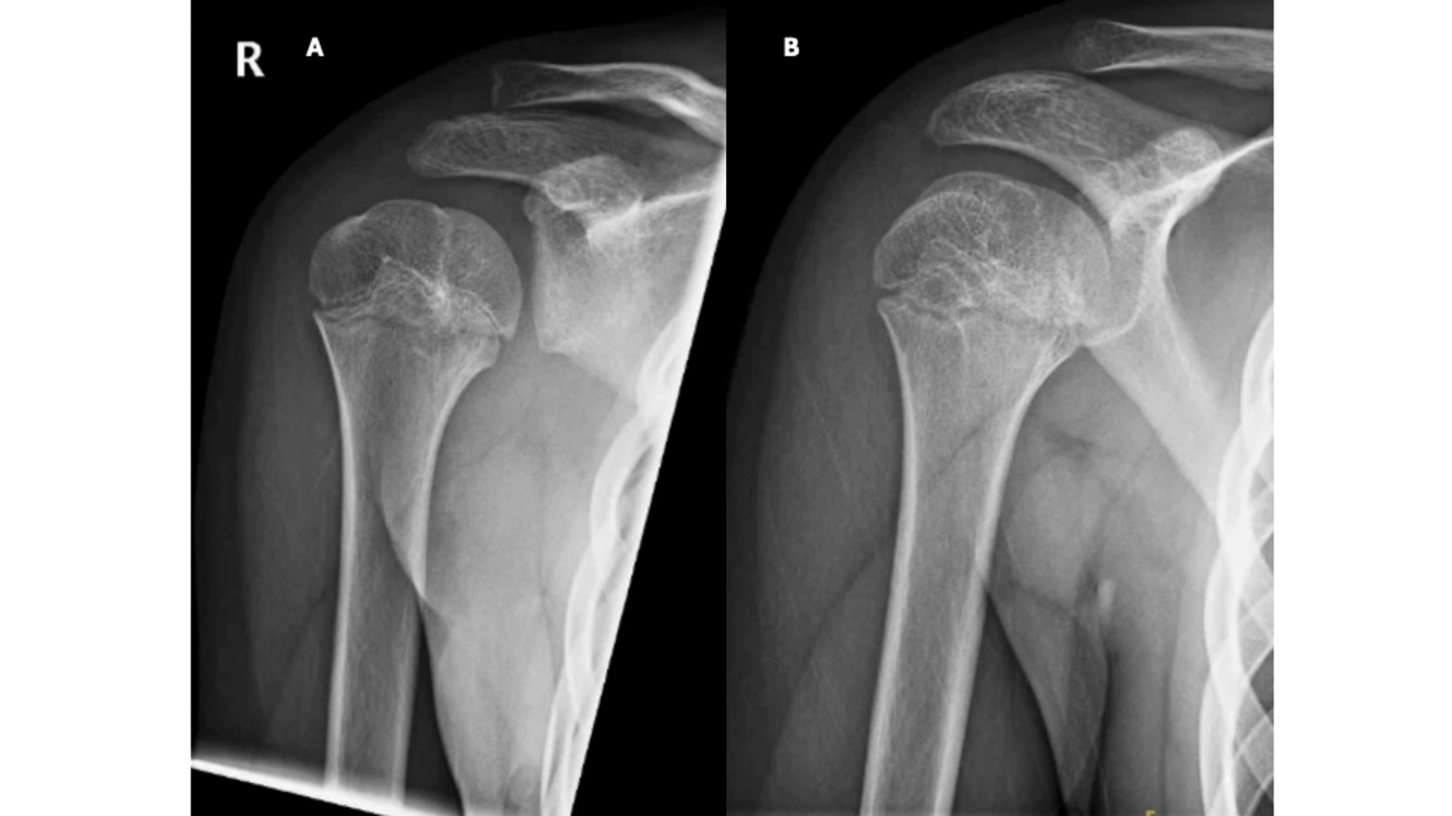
Follow-up radiographs showing a united fracture with no signs of avascular necrosis at 12 months. (A) Grashey and (B) anteroposterior (AP) views are shown.
